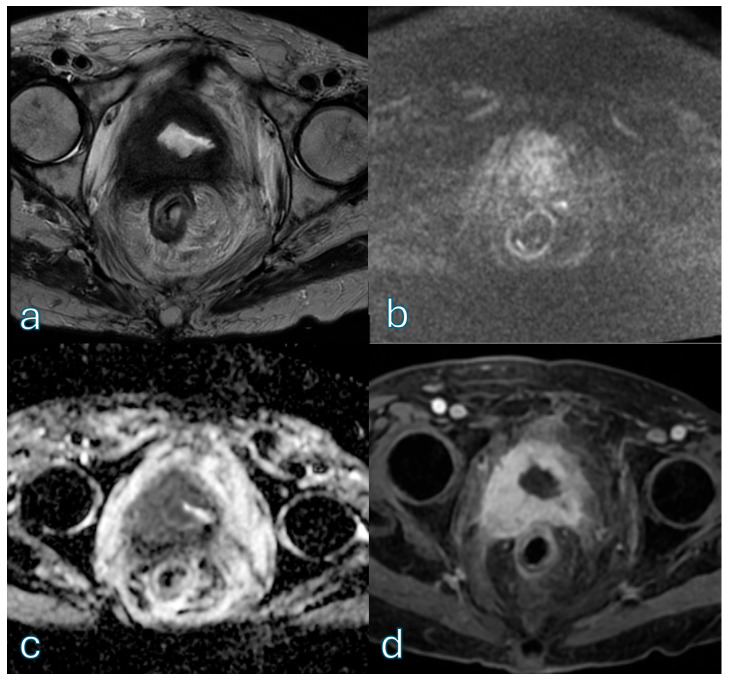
Multiparametric MRI demonstrates a post-TURBT bladder with intraluminal blood products, resulting in marked bladder wall irregularity and thickening. On T2-weighted imaging (a), postoperative changes and hemorrhagic content obscure normal wall stratification, a pattern that could lead to misclassification as VI-RADS 5 if assessed without contrast enhancement. However, diffusion-weighted imaging/ADC maps (b,c) do not show convincing focal diffusion restriction suggestive of viable tumor infiltration. Importantly, dynamic contrast-enhanced (d) imaging demonstrates absence of early tumoral enhancement and preservation of the muscular layer, allowing correct interpretation of postoperative changes rather than muscle-invasive disease. This case highlights the critical role of multiparametric MRI in the post-TURBT setting and the limitations of bpMRI-only assessment, where the risk of clinically relevant overstaging is substantial.
